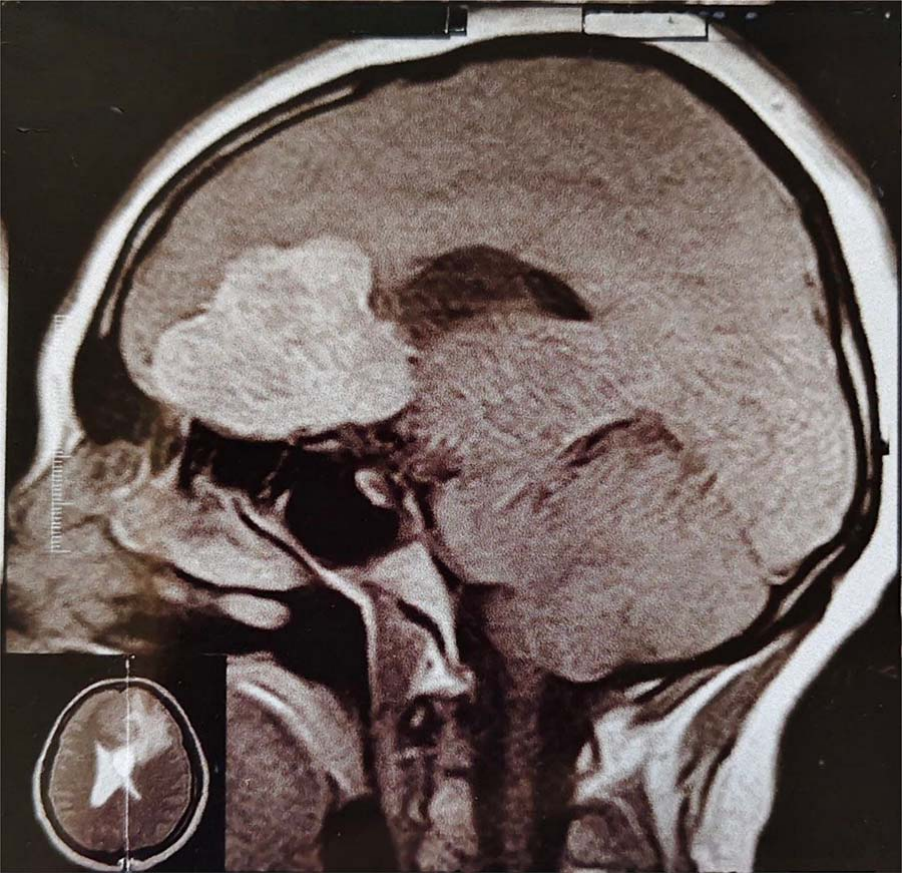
MRI brain with IV contrast (sagittal midline view) showing a homogeneously enhancing mass based on the cribriform plate of the ethmoid, displacing the frontal lobe upward and posteriorly.
